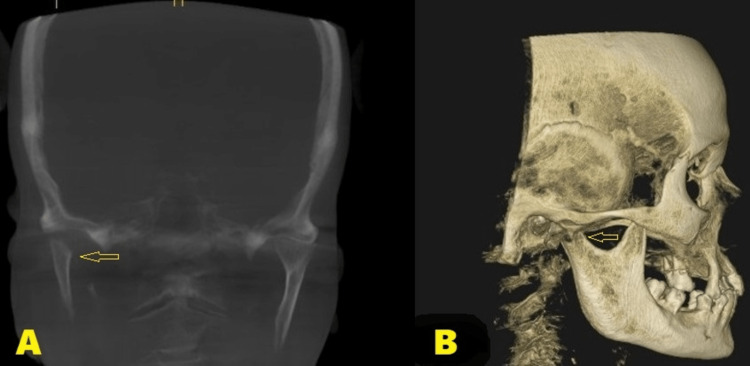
Postoperative CBCT scan Postoperative cone beam computed tomography (CBCT) scan showing good wound healing (arrows) in A) Coronal section B) Three-dimensional sagittal view
